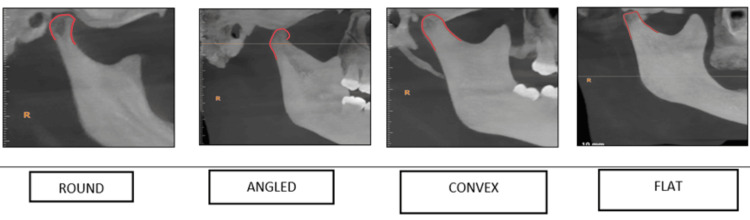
Tracing of the morphological variations of the condyle in CBCT images CBCT: cone beam computed tomography
